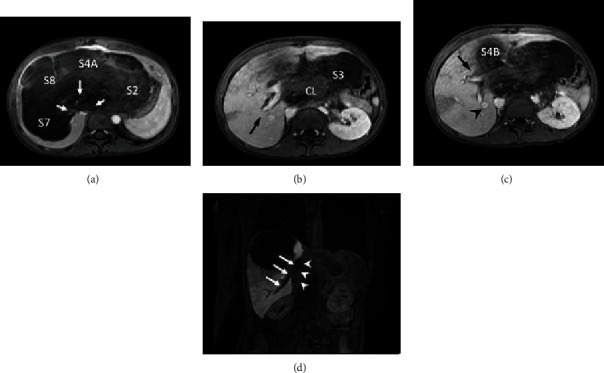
MRI Images. (a) Axial T1 weighted: tumor involvement of major hepatic veins (arrows) and liver segments 2, 4A, 7, and 8. (b) Axial T1 weighted: tumor involvement of segment 3 and caudate lobe; right posterior portal vein is free (arrow). (c) Axial T1 weighted: tumor involvement of segment 4B and the right anterior portal vein (arrow); IRHV entering segment 6 (arrowhead). (d) Coronal T1 weighted, 20-minute hepatobiliary phase: view of the IRHV in segment 6 (arrows) draining into the IVC (arrowheads).
